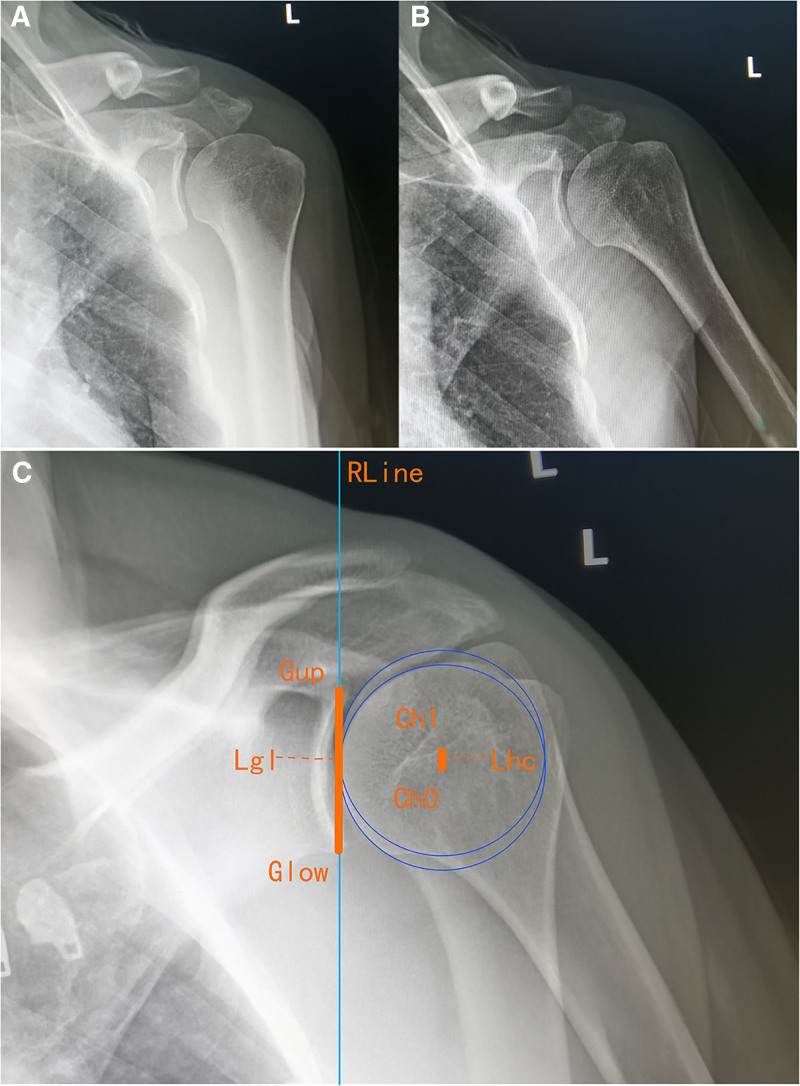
An image-based measurement technique for the evaluation of the upward movement of the humeral head. Anteroposterior (AP) X-rays of the shoulder joint in abduction positions of 0° (A) and 30° (B). (C) The calculation of the relative magnitude of the humeral head’s upward movement (Rlhg = Lhc/Lgl).
